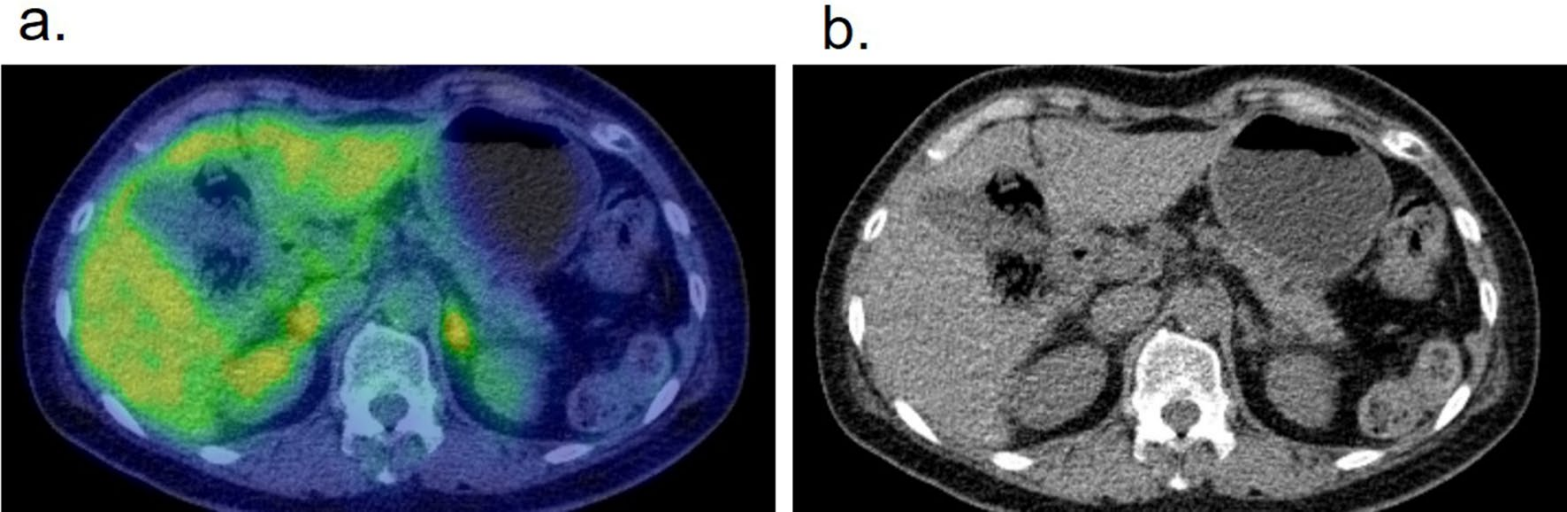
Increased FDG uptake in both adrenal glands with normal configuration in a case who developed vasovagal reflex at the FDG injection. a Fusion image, b CT
